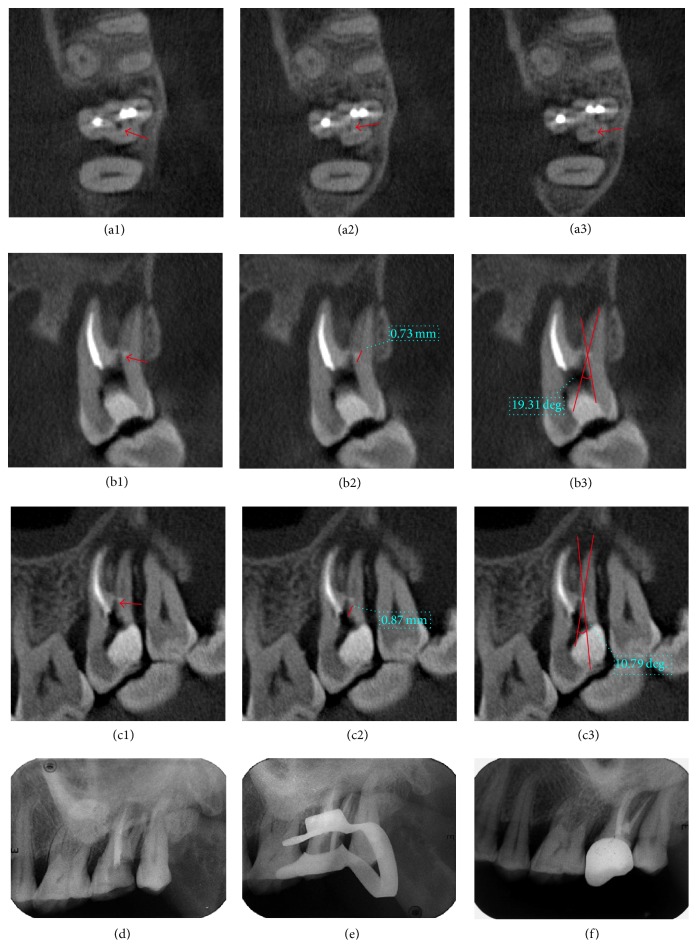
Case  3: (a1)–(a3) distobuccal canal managed by ultrasonic instrument before CBCT scanning (red arrow). (a2) Calcification of palatal canal (red arrow). (a3) Canal under calcification (red arrow). (b1)–(b3) Determination of depth in buccolingual direction and BLIA. (c1)–(c3) Determination of depth in mesiodistal direction and MDIA. (d) X-ray radiograph before operation. (e) Confirmation of working length. (f) Canals after filling and restoration.
